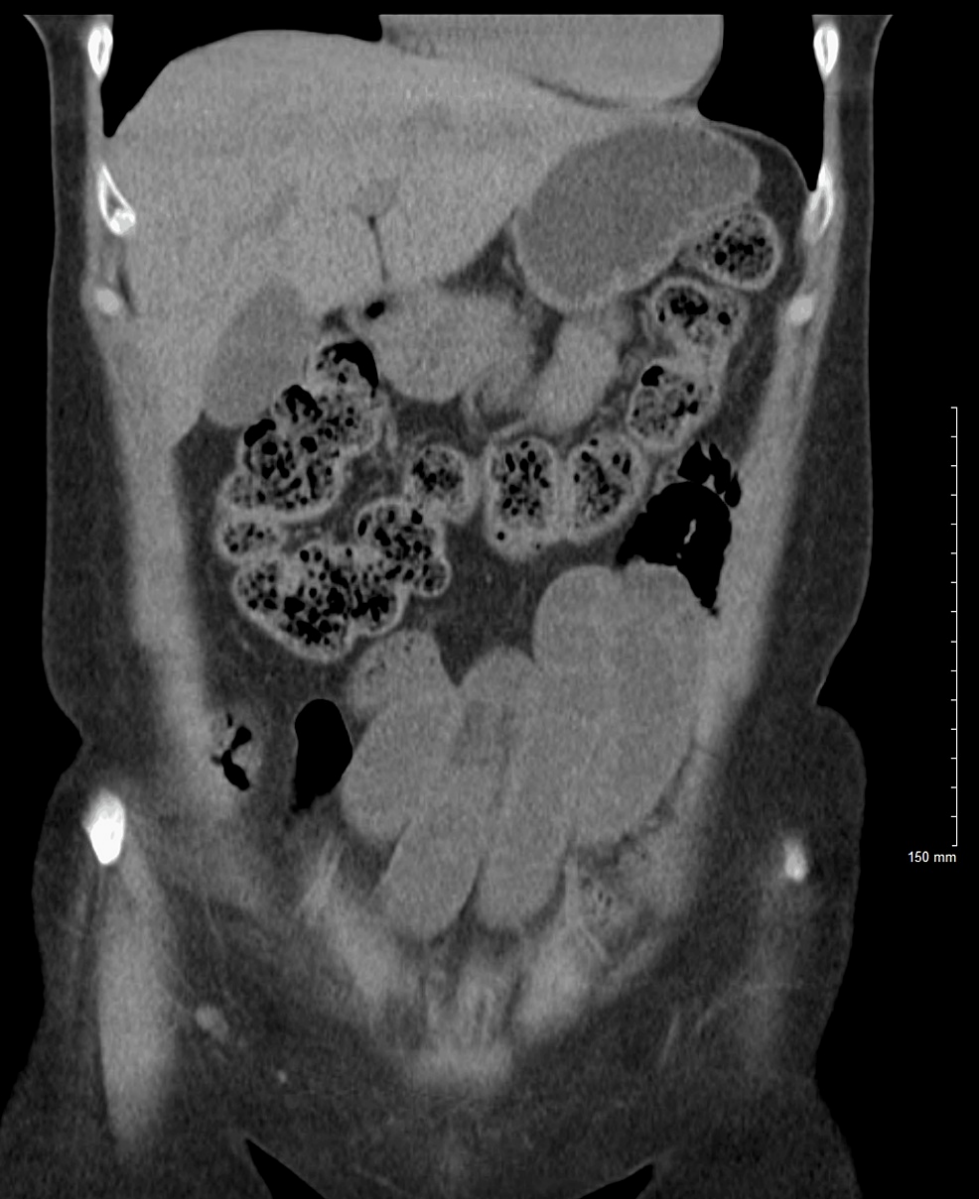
Coronal non-contrast CT of the abdomen and pelvis with no acute findings.
